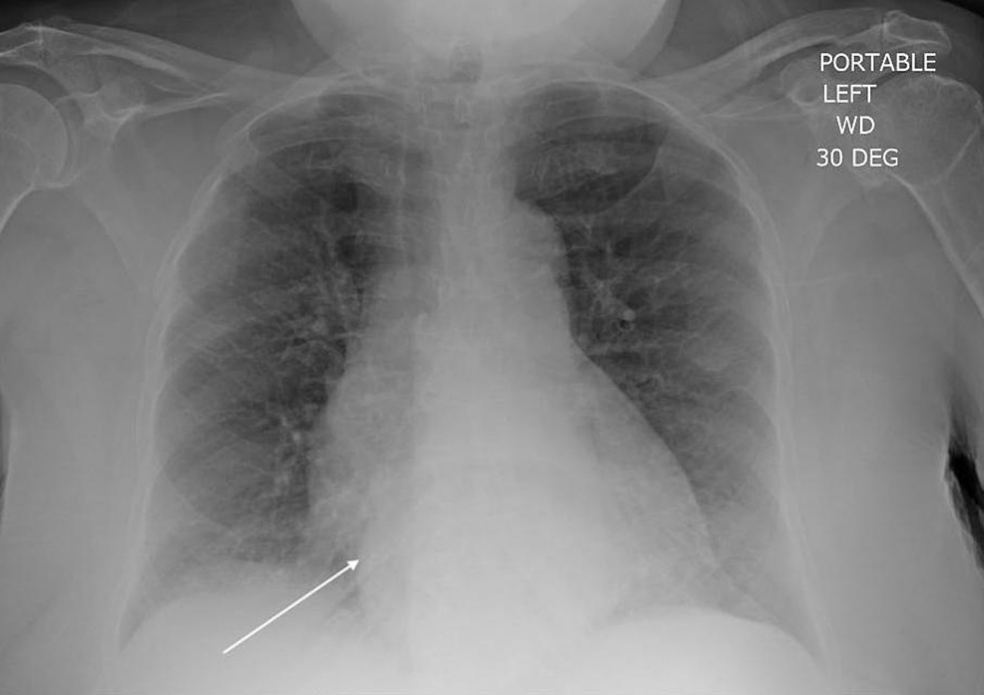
Chest x-ray showing outline of retrocardiac opacity and mild interstitial ground glass opacities bilaterally. Arrow demonstrates the extrinsic compression of the left atrium by the giant hiatal hernia.
